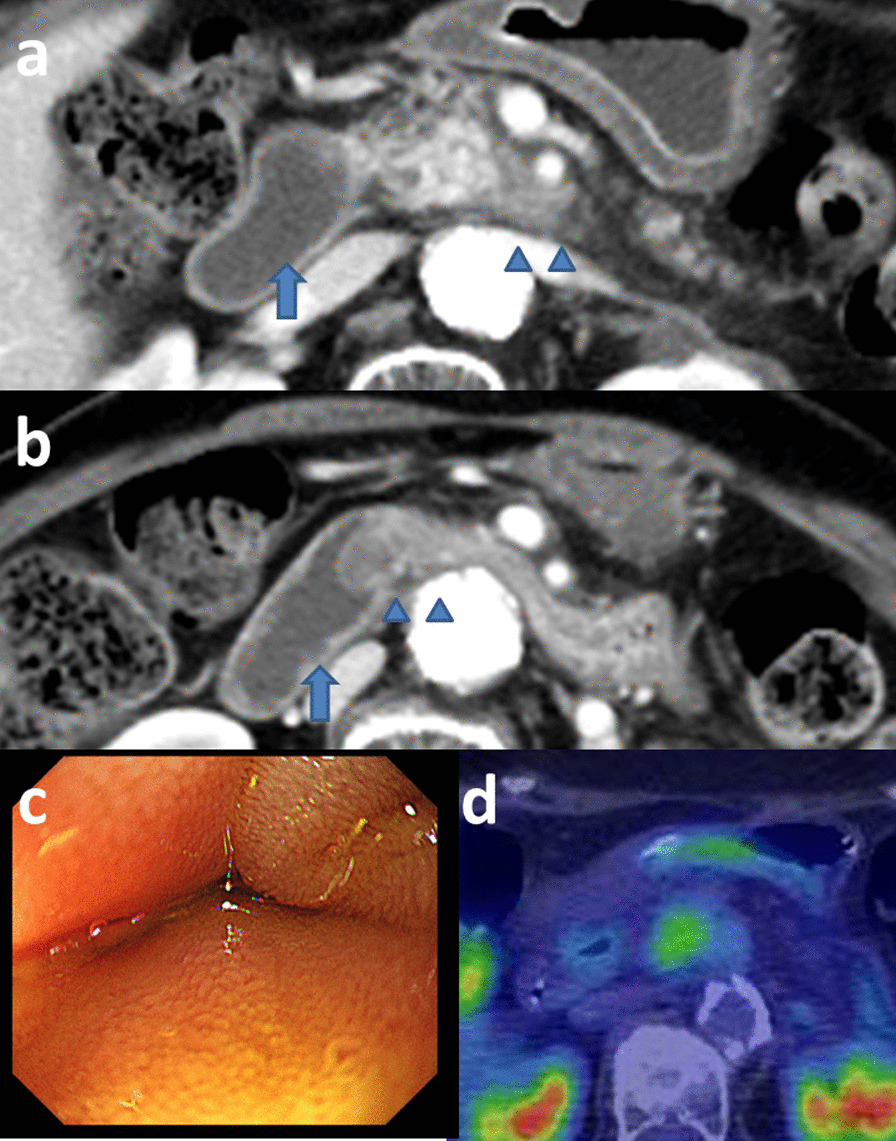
Findings of imaging studies after carbon-ion radiotherapy. a Contrast-enhanced computed tomography revealed no tumor growth (arrowheads). However, duodenal dilatation was observed (arrow). b CECT revealed a duodenal obstruction accompanied by wall thickening at the third portion of the duodenum (arrowheads). The oral duodenum was dilated (arrow). c Endoscopy revealed a duodenal compressive obstruction. The endoscope could not pass through this duodenal obstruction. d 18-fluoro-2-deoxy-glucose positron emission tomography/computed tomography after carbon-ion radiotherapy revealed slight FDG accumulation (standardized uptake value ranging from 3.14 to 3.20) in the pancreatic lesion without findings of distant or lymph nodal metastasis
